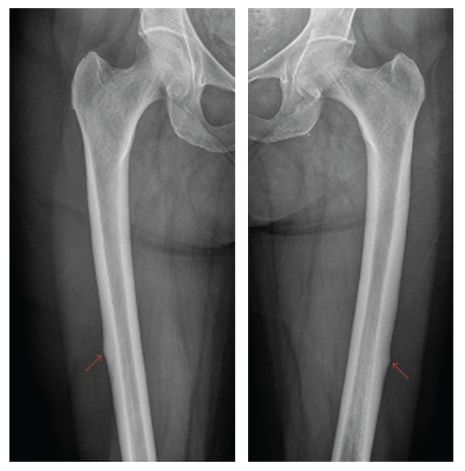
Radiograph of both femurs showing stress fracture and lateral cortical thickening.
